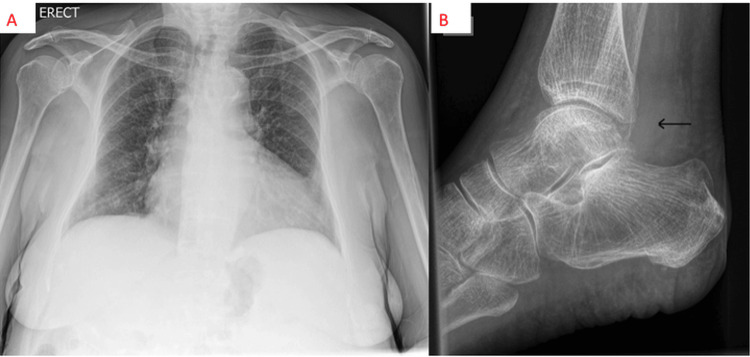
(A) Initial chest X-ray at presentation - lungs appeared clear. (B) Plain radiograph of the left ankle (horizontal beam view). Note the superadded density in the posterior recess of the ankle joint (line arrow), corresponding to synovitis and effusion. No cortical erosion at the time of imaging.
